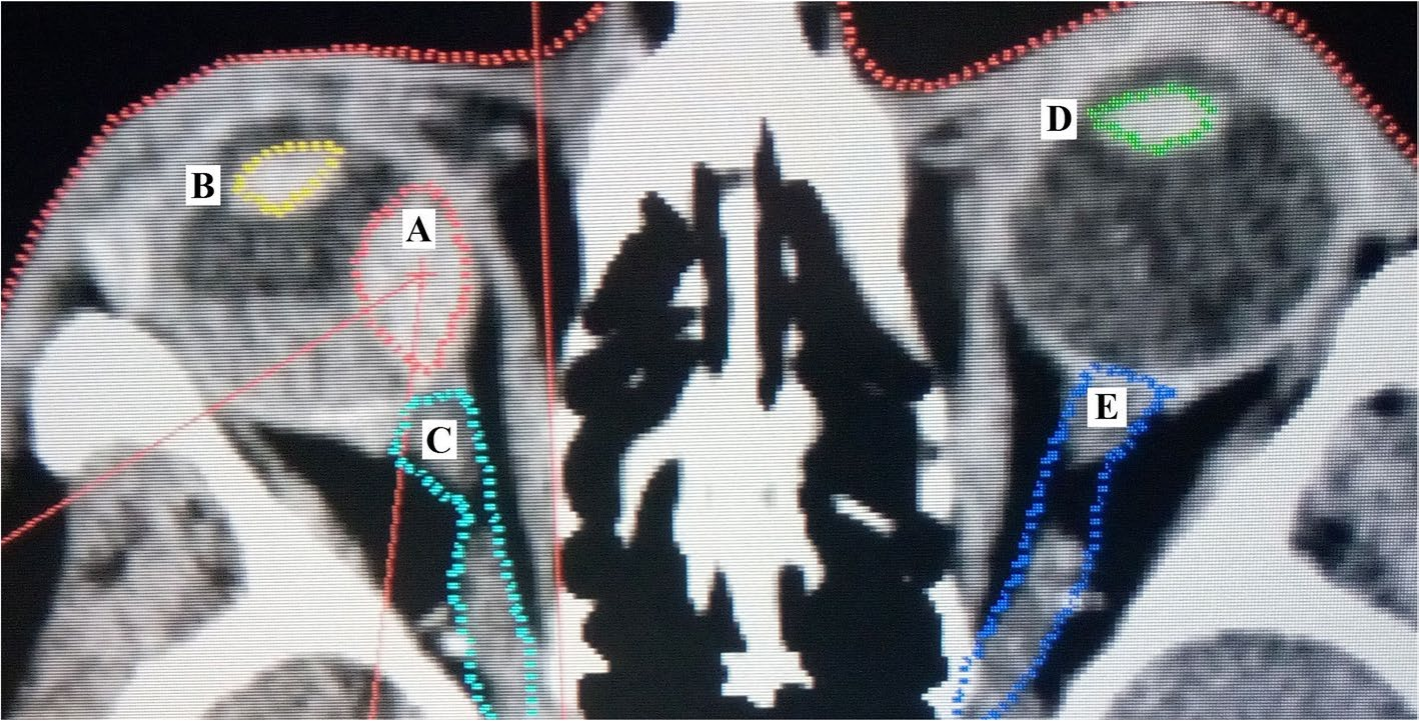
Stereotactic planning scheme - fused CT with MRI findings’ scans of the patient with uveal – choroidal melanoma at the day of stereotactic irradiation: A – tumor, B – lens of the irradiated eye globe, C - optic nerve of the eye, with uveal tumor, D - contralateral lens, E - contralateral optic nerve (Source: Embedded planning software of LINAC C 600 C/D Varian located at the workplace of the authors)
